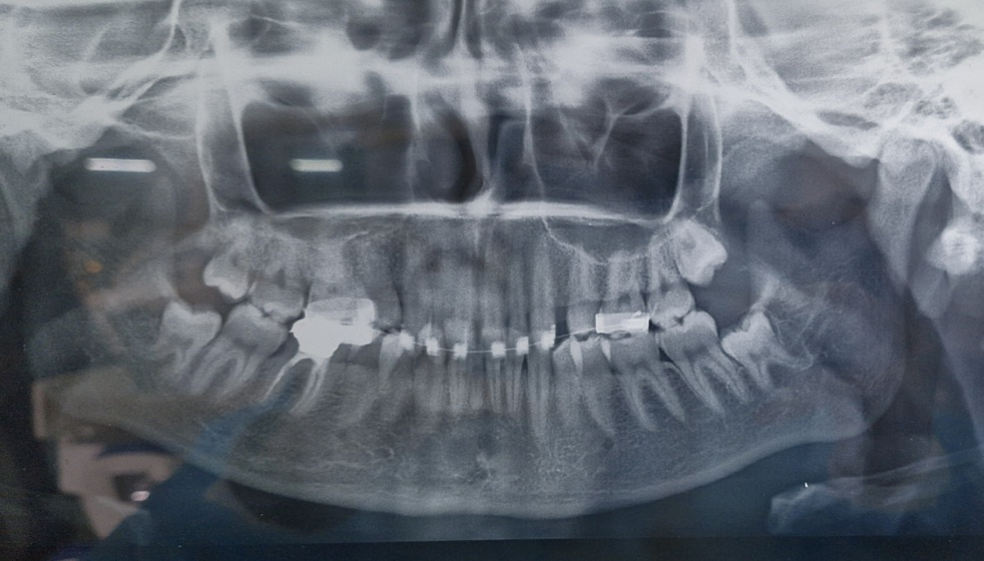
Pretreatment orthopantomogram.
